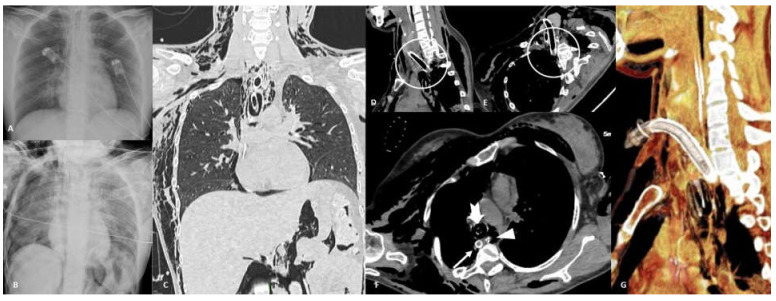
Perforation. Thirty-six-year-old female affected by acute leukemia who underwent tracheostomy placement. Frontal chest radiograph before (A) and after (B) tracheostomy procedure show the development of right pneumothorax, pneumomediastinum, pneumoperitoneum, and soft tissue emphysema onset. Coronal non-contrast CT scan in lung window (C) confirms all these findings. CT unenhanced multiplanar reconstructions (D,E) show posterior tracheal wall perforation induced by the tip of the tracheostomy tube; CT axial image (F) shows displacement of tracheostomy tip in the posterior mediastinum/prevertebral soft tissue (arrow), correct position of nasogastric tube (NSG) in esophageal lumen (arrowhead), and endotracheal tube correctly cuffed (notched arrow). CT 3D-reconstruction (G) better shows tracheostomy tube malposition.
